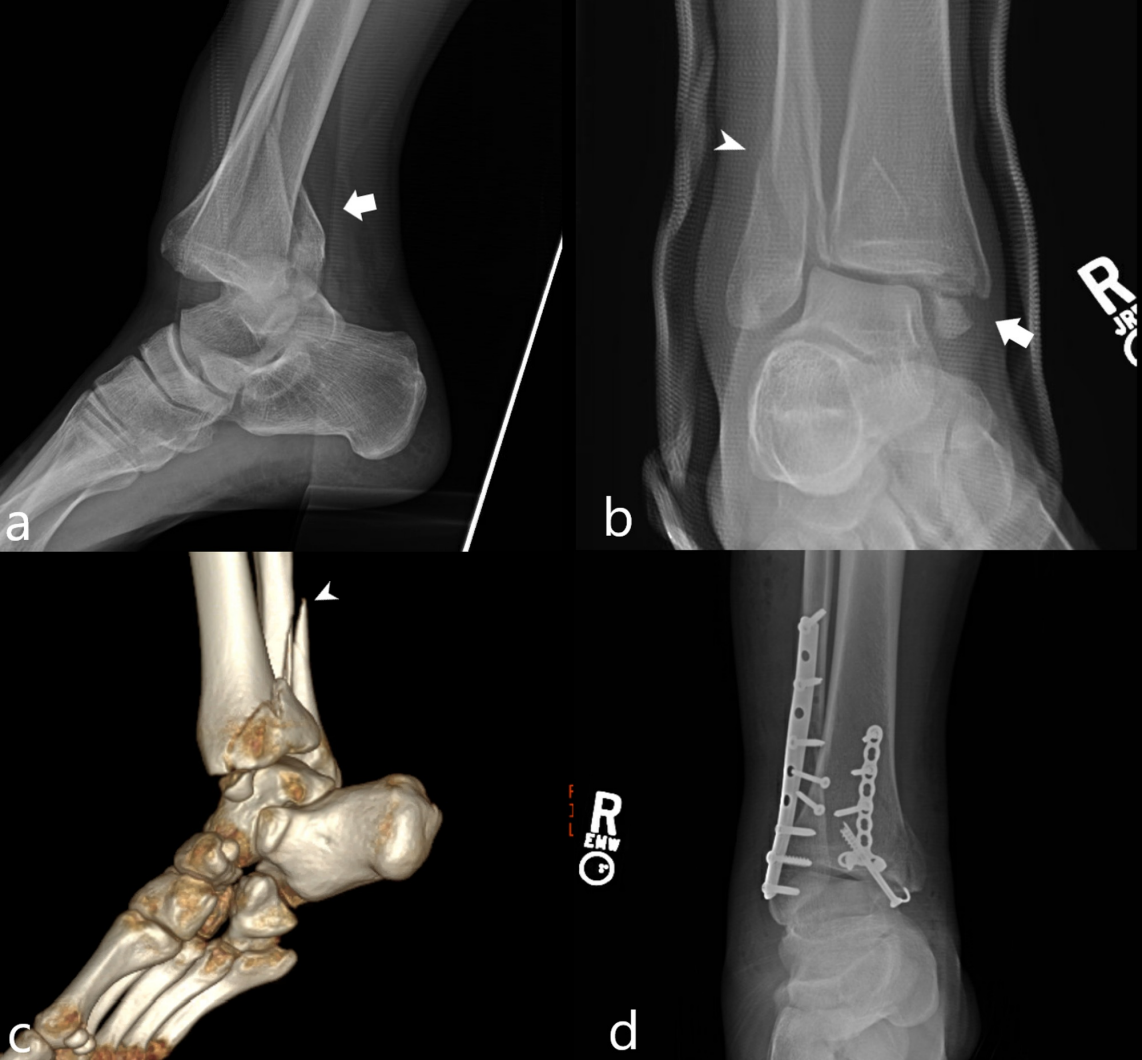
Case 6 radiologic images A lateral radiograph of the ankle (a) demonstrates tibiotalar joint dislocation as well as a fracture of the posterior malleolus (white arrow). A frontal radiograph of the ankle (b) demonstrates a nondisplaced fracture of the lateral malleolus (white arrowhead) and a moderately displaced fracture of the medial malleolus (white arrow). A reconstructed 3D image (c) demonstrates the lateral malleolar fracture to a better degree. A post-operative frontal radiograph (d) demonstrates open reduction/internal fixation of the trimalleolar ankle fracture.
